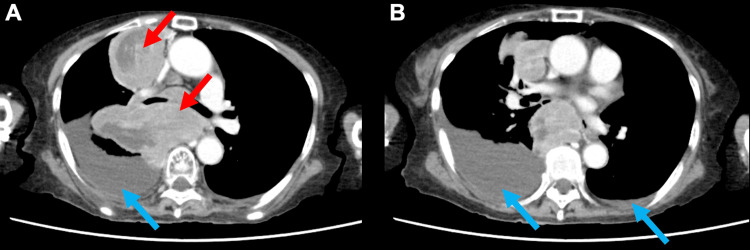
Initial chest CT image showing a bulky mediastinal tumor and bilateral pleural effusions Figure 1A shows a bulky mediastinal tumor (red arrows) compressing the esophagus. Figure 1A and Figure 1B demonstrate bilateral pleural effusions, which are more predominant on the right side (blue arrows). CT: computed tomography
